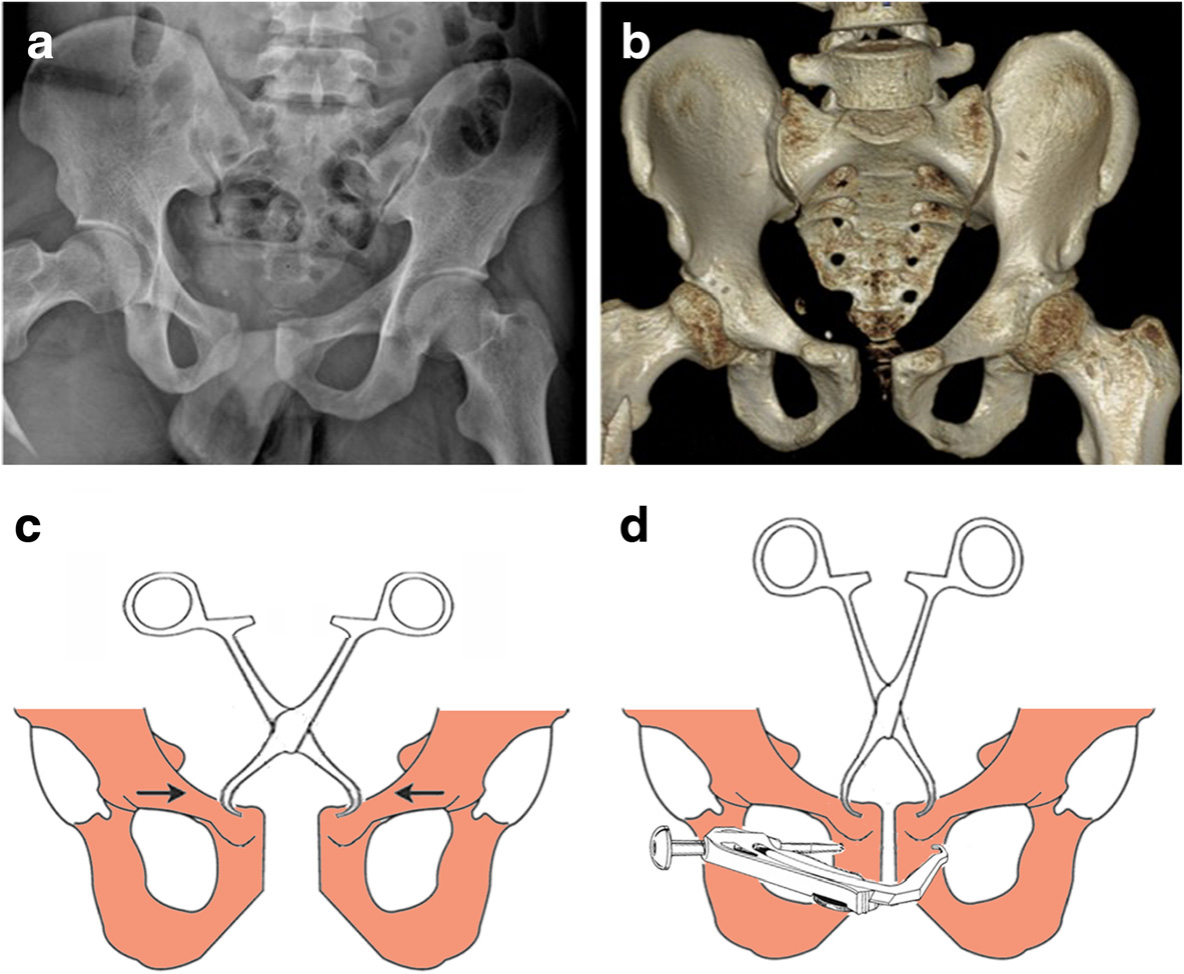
A male patient suffered pubic symphysis diastasis in a road traffic accident. a Preoperative anteroposterior radiograph. b Three-dimensional CT. c Reduction is maintained using pointed reduction clamps. d The insertion and exertion of the guide wire are located using a drill guide
